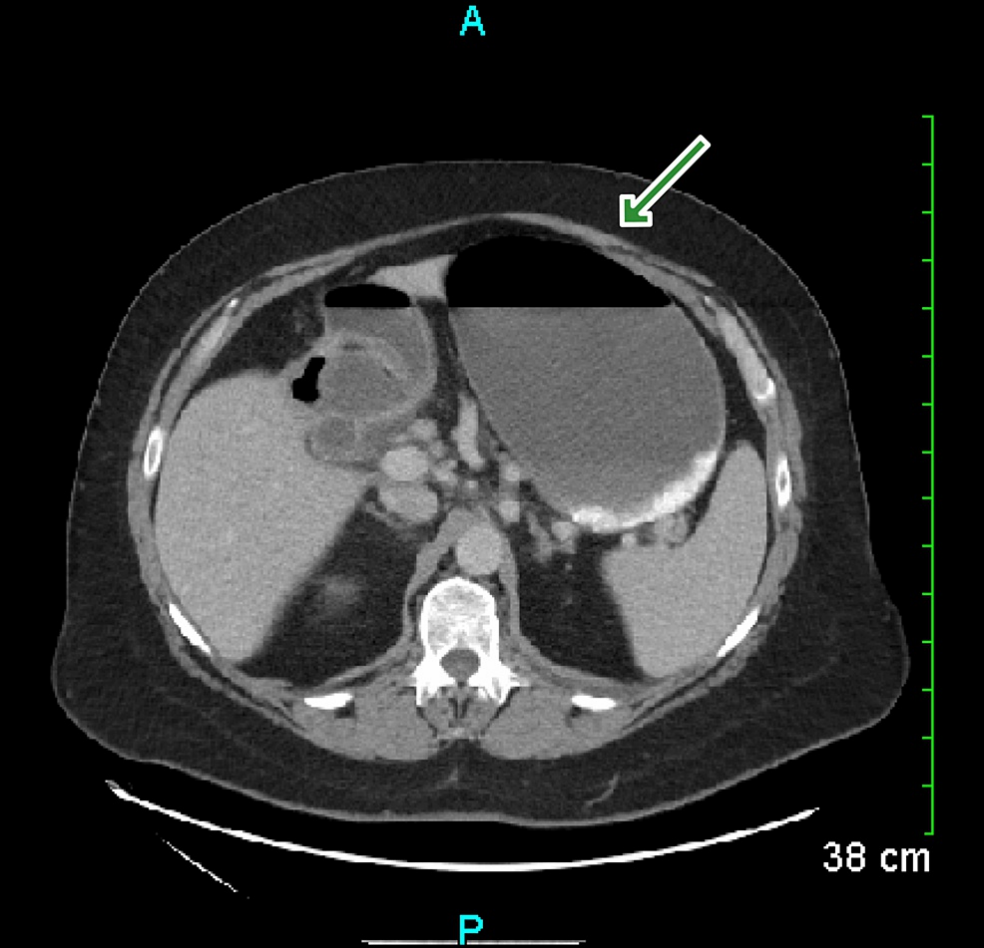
CT abdomen Green arrow pointing towards a severely distended stomach with air-fluid level.
